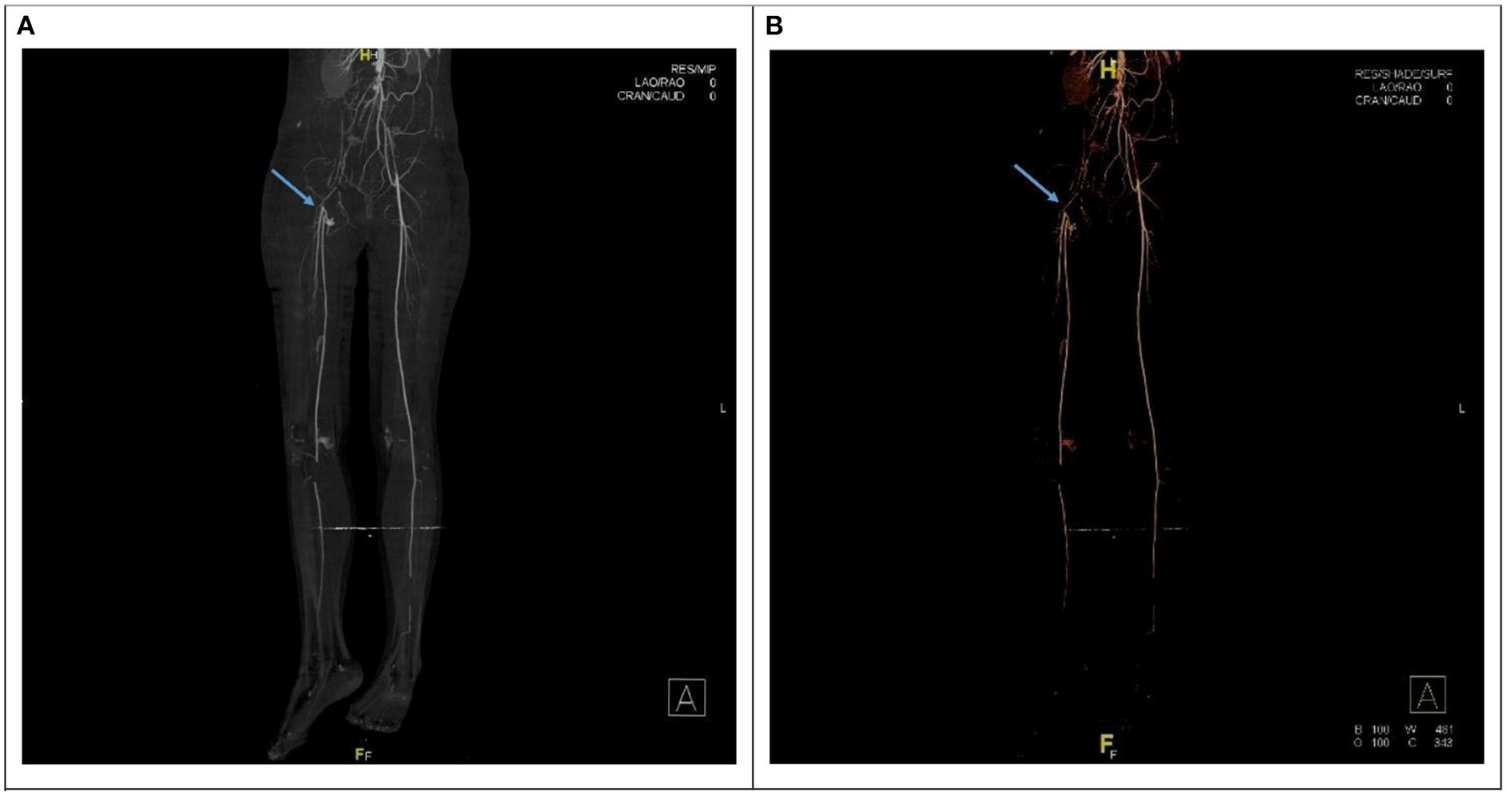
(A,B) CT angiogram showing interruption of the contrast at the aorto-iliac arterial level correlated with embolization at the aortic bifurcation (saddle embolus) with thrombus propagation. The blud arrow point to no visualization of the right external iliac artery.
